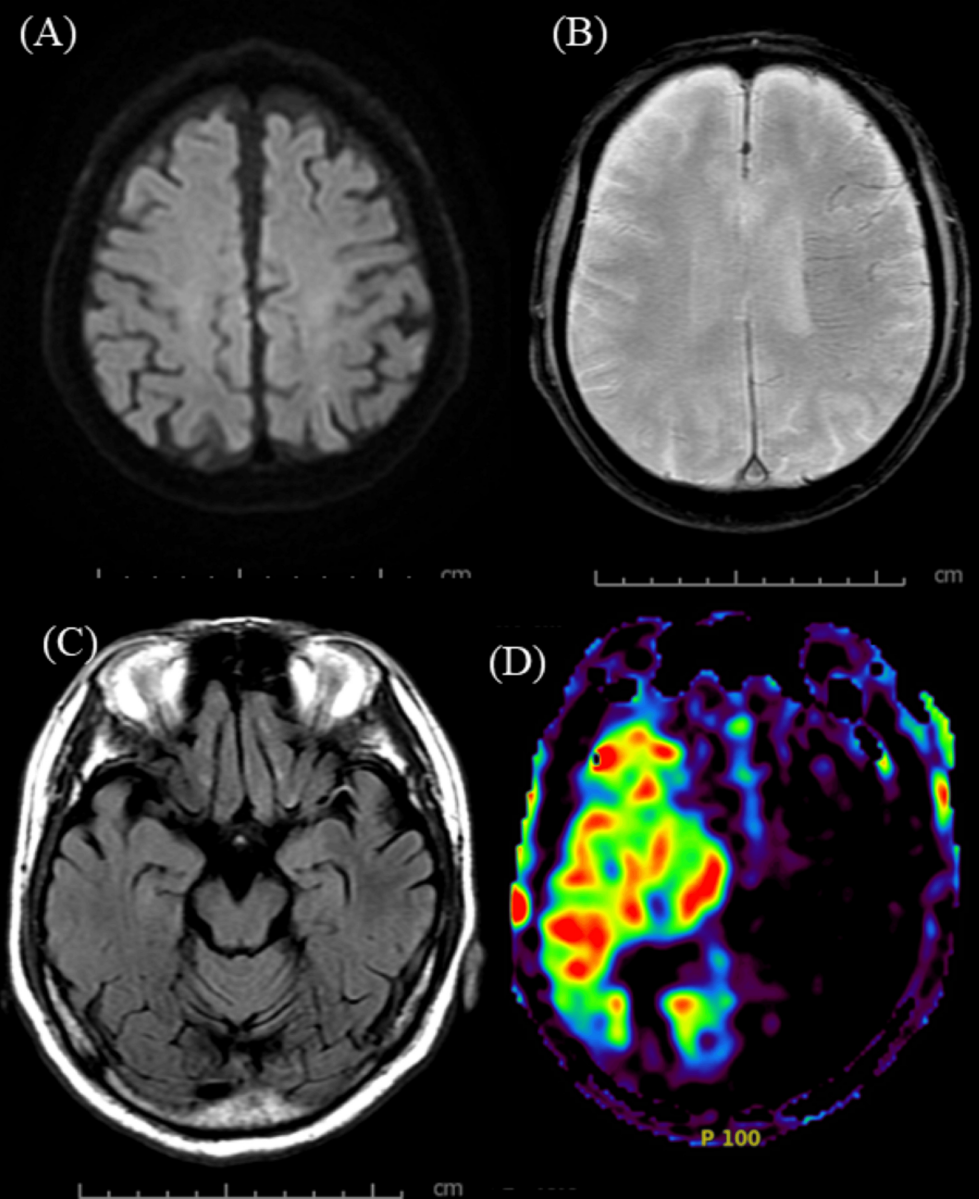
Preoperative magnetic resonance imaging (MRI) findings. (A) Diffusion-weighted imaging (DWI) reveals faint hyperintensity in the left parietal lobe. (B) T2*-weighted imaging demonstrates the brush sign along the periventricular medullary veins in the left hemisphere. (C) Fluid-attenuated inversion recovery (FLAIR) imaging shows linear hyperintensity (intra-arterial sign) within the left middle cerebral artery (MCA). (D) Arterial spin labeling (ASL) indicates extensive hypoperfusion in the left cerebral hemisphere.
